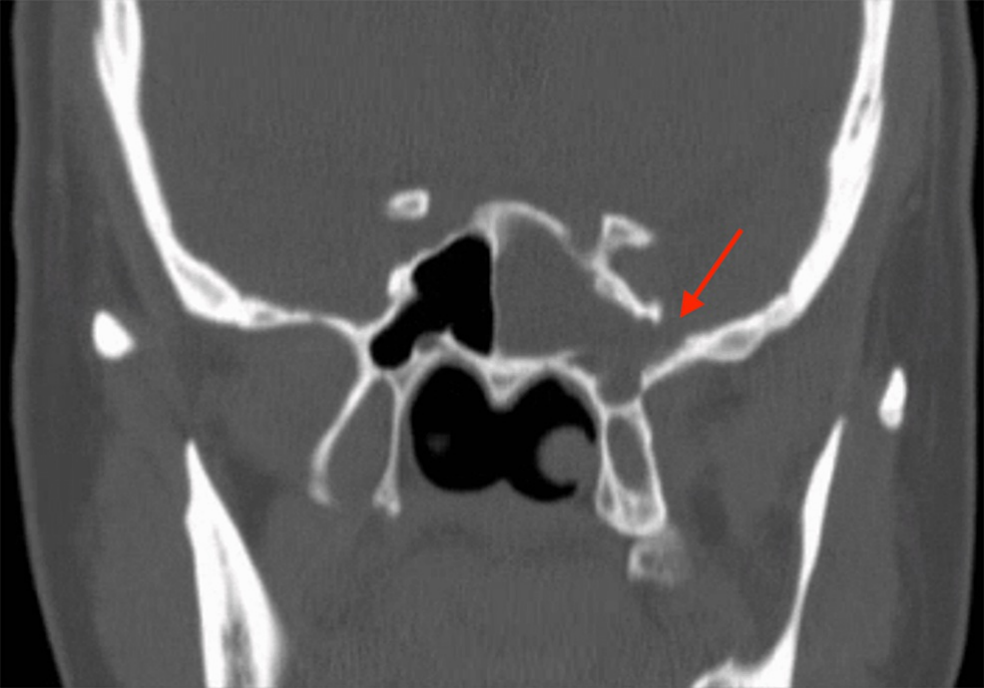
Coronal computed tomography (CT) of the head and sinus As depicted by the red arrow, the coronal CT of the head and sinus showed soft tissue in the lateral aspect of the left sphenoid sinus that appears to communicate with the inferior aspect of the left temporal lobe through a bone defect.
